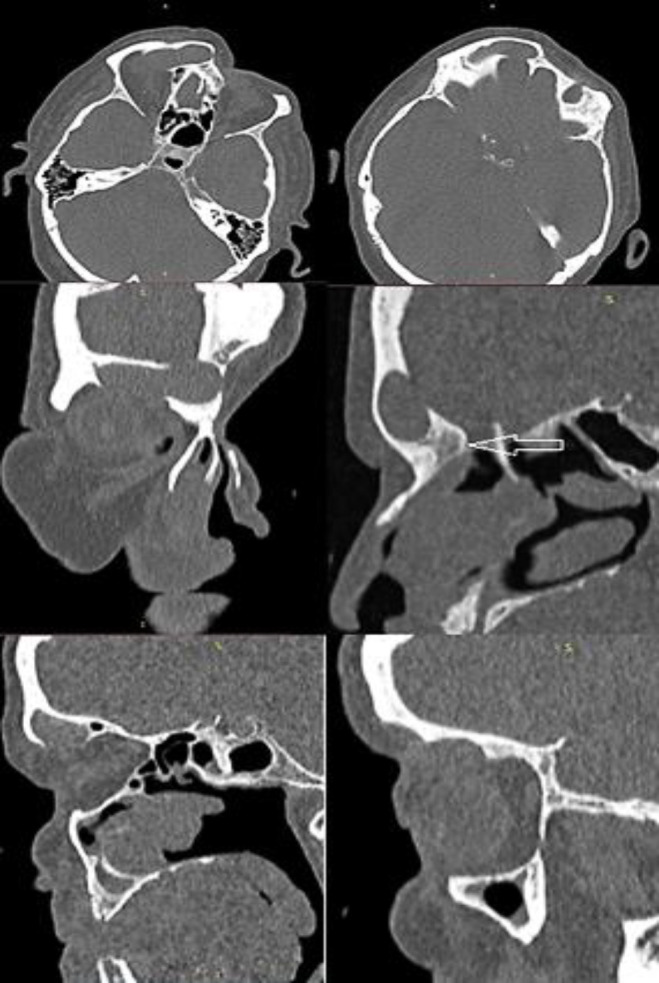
Paranasal sinus CT scan showing a 23×10×10 (height-length-width) mm bony mass (white arrow) arising from the right frontoethmoidal area which causes total opacification of the right frontal sinus, inferior and posterior sinus wall defect
